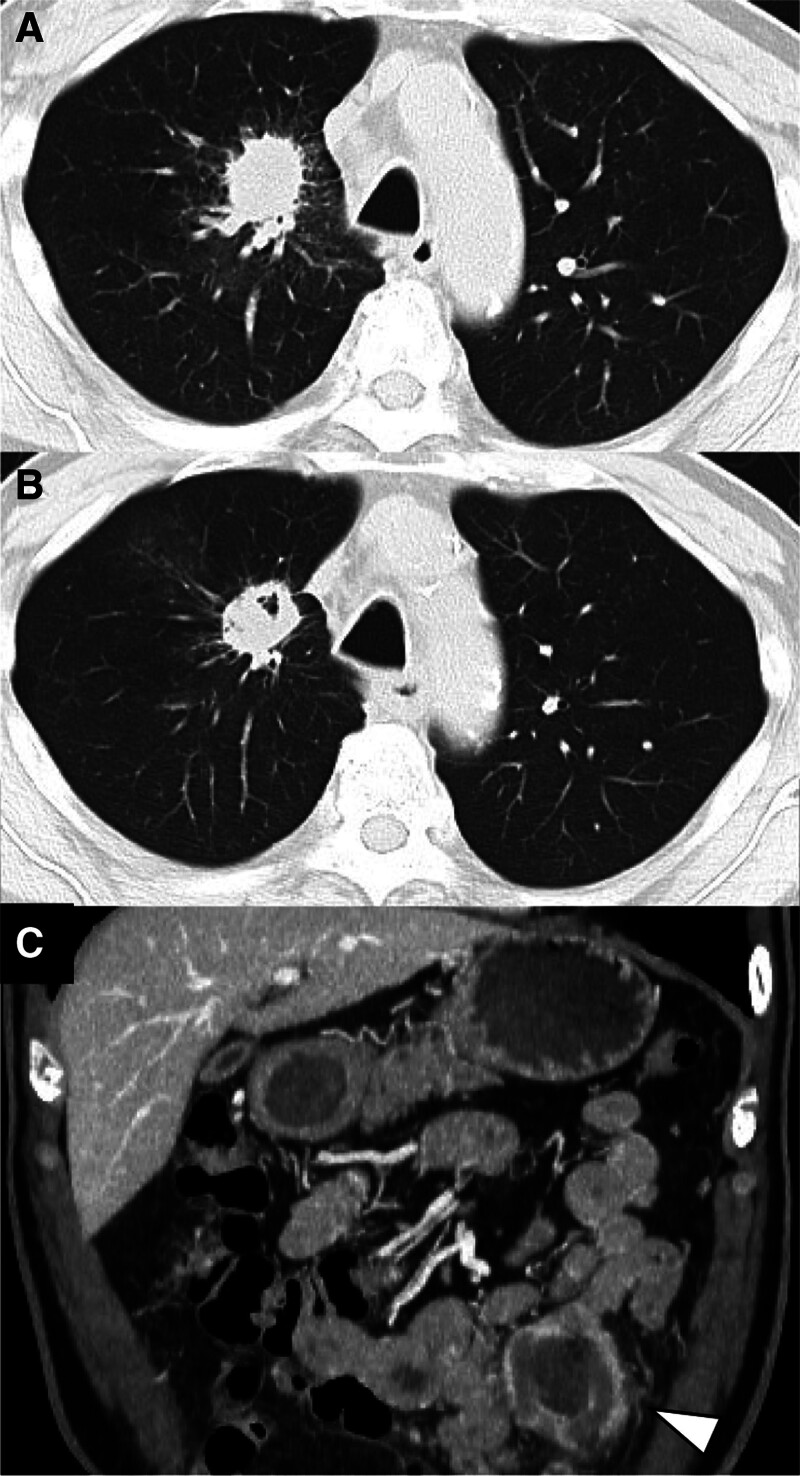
(A) Initial chest computed tomography (CT) image showing a 70 mm lesion in the right pulmonary hilum. (B) Chest CT image after completion of chemoradiotherapy (CRT) showing tumor reduction. (C) Abdominal CT image obtained two weeks after completion of CRT showing thickened small-bowel walls (arrowhead), considered suspicious for metastasis.
